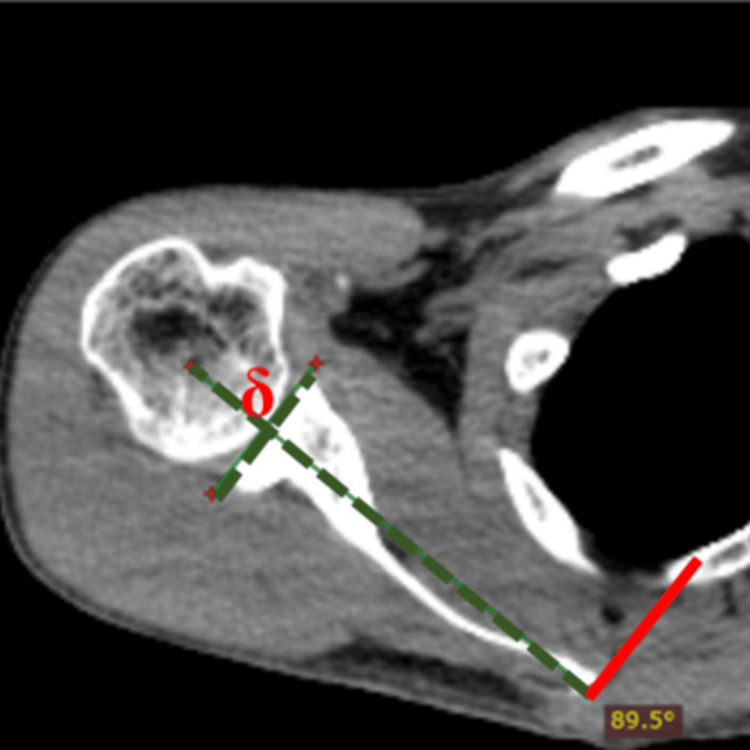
2D axial CT image of the right scapula showing glenoid version angle measurement The first line is drawn from the mid-point of the glenoid with the vertebral border of the scapula, and the second line is drawn from the anterior and posterior margins of the glenoid; the angle subtended by these two lines was marked angle δ CT: computed tomography
